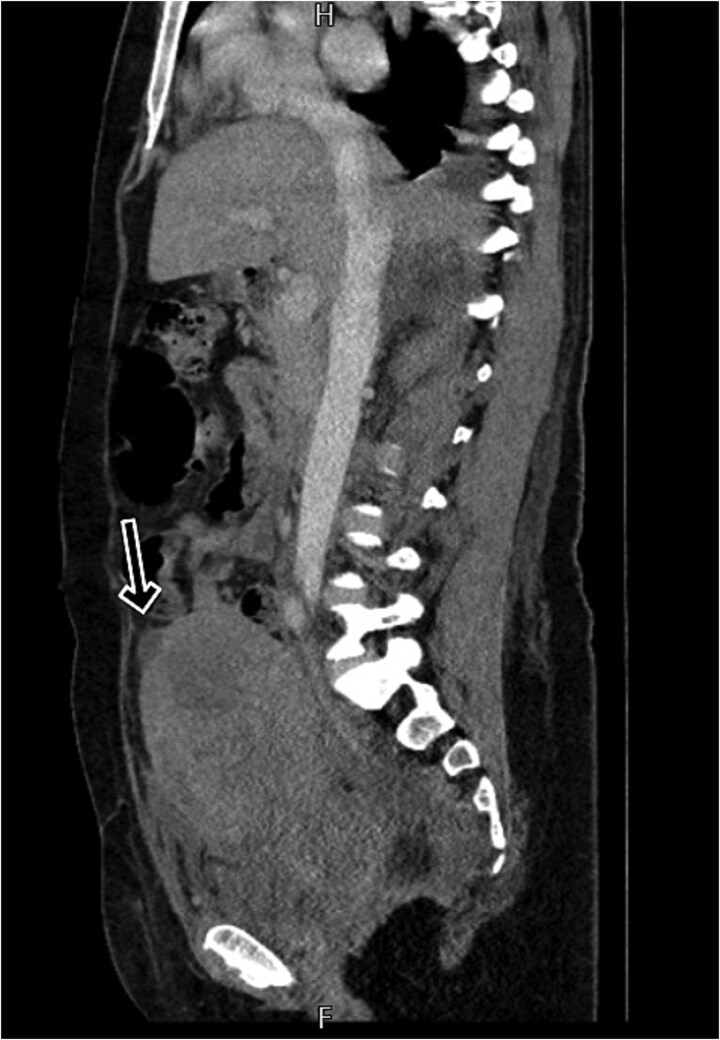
CT scan shows a linear small defect in the anterior wall of uterine fundus with small collection (2 × 1 cm).
